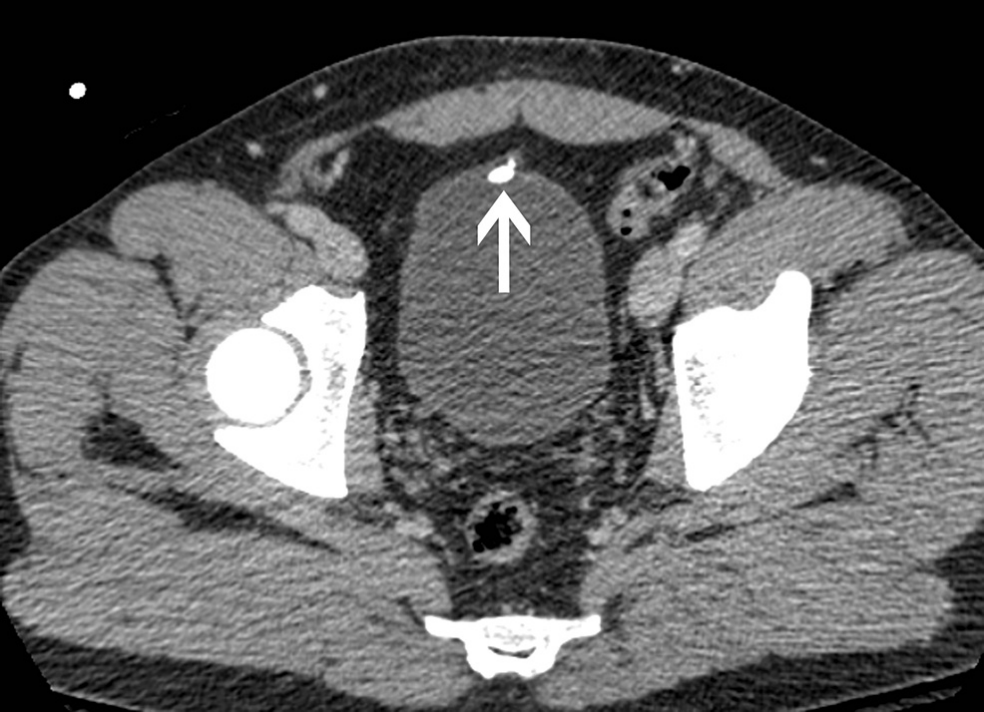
Axial CT image throughout the pelvis shows a 7 mm calcification (arrow) attached to the midline of the anterior wall of the urinary bladder.
